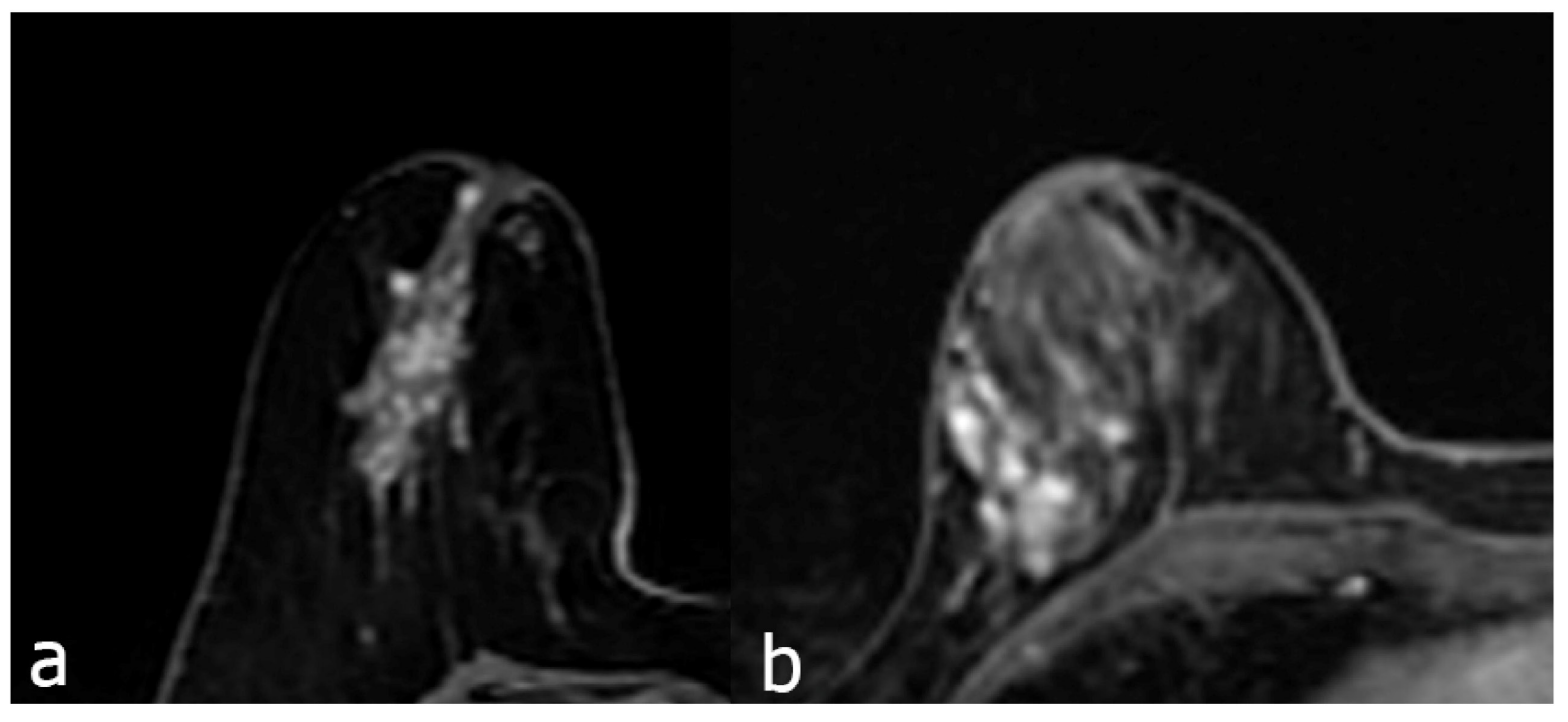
The first contrast enhanced breast MRI image (a), 49 years old, is a segmental heterogeneous enhancing NME lesion, and the pathology result is adenosis. Second image (b), 41 years old, regional heterogeneous enhancing NME lesion, biopsy result is invasive carcinoma.
